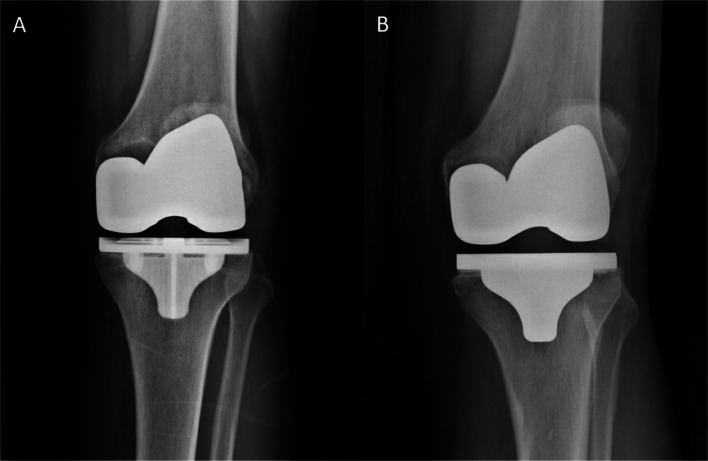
Representative anteroposterior postoperative radiographs of cementless (A) and hybrid (B) total knee arthroplasty showing radiolucent lines at the bone–implant interface
